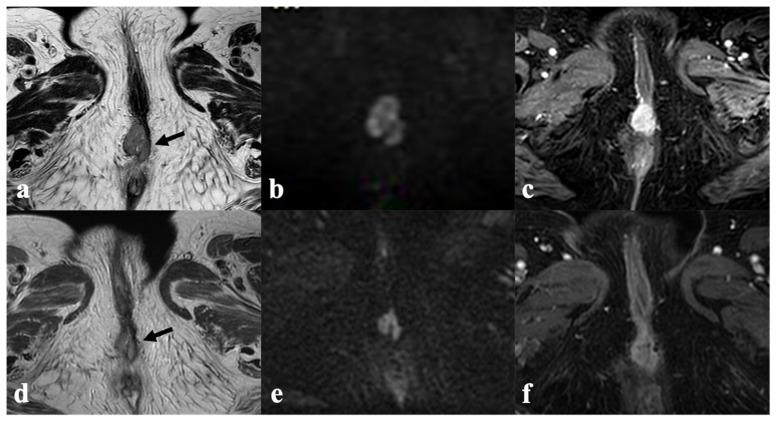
Partial response to RT, in recurrent vulvar cancer. A 79-year-old patient with recurrent vulvar cancer after partial vulvectomy (upper row) presenting with an intermediate T2 signal intensity mass in the right vulvar region ((a)—black arrow), with hyperintensity in DWI (b) and enhancement in T1-WI post-contrast (c). MRI after CRT (bottom row) shows lesion size reduction in both T2W ((d)—black arrow), DWI (e) and post-contrast T1W images (f) consistent with partial response.
